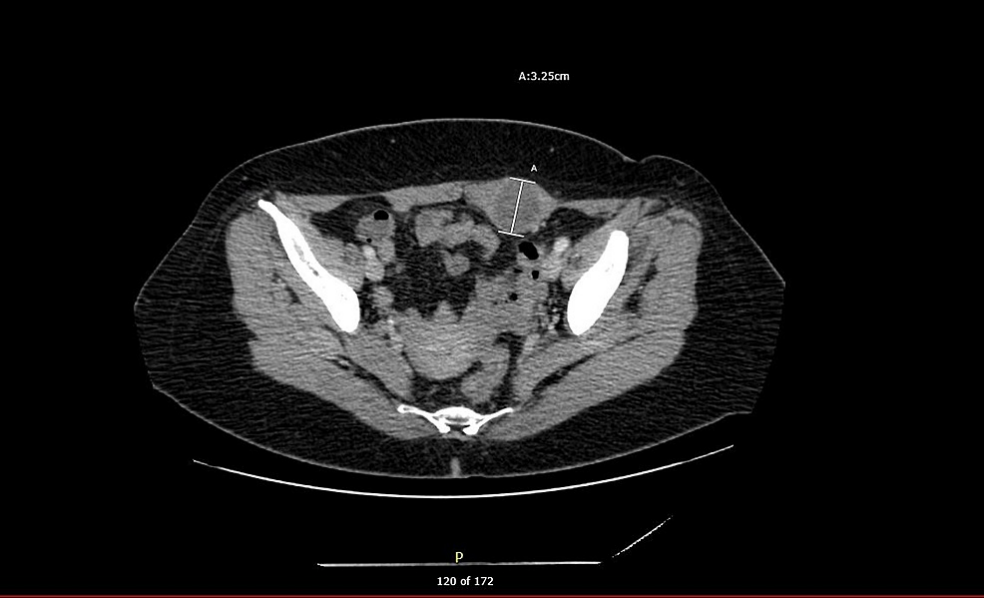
CT abdomen/pelvis with contrast for surveillance showing a 3.25 cm lesion in the left rectus abdominis
